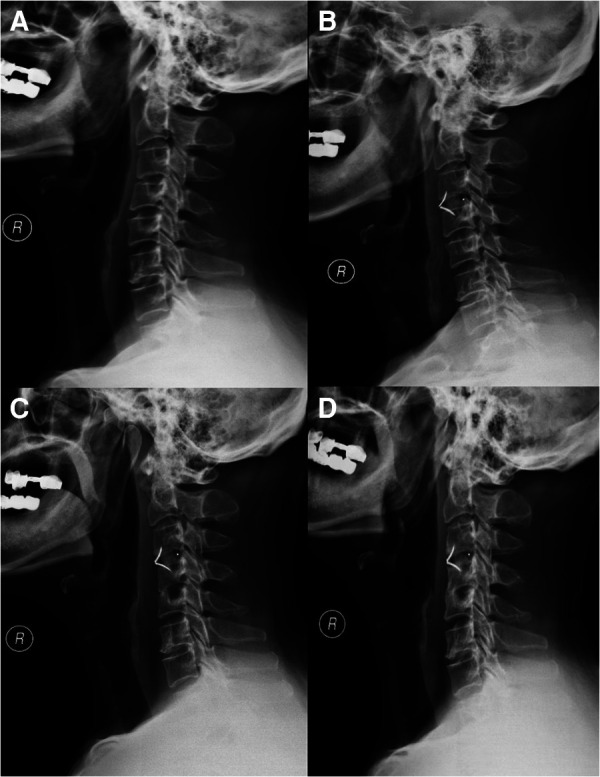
(A) X-ray before operation. (B) X-ray 2 days after the operation. (C) X-ray 2 months after the operation. (D) X-ray 12 months after the operation.
